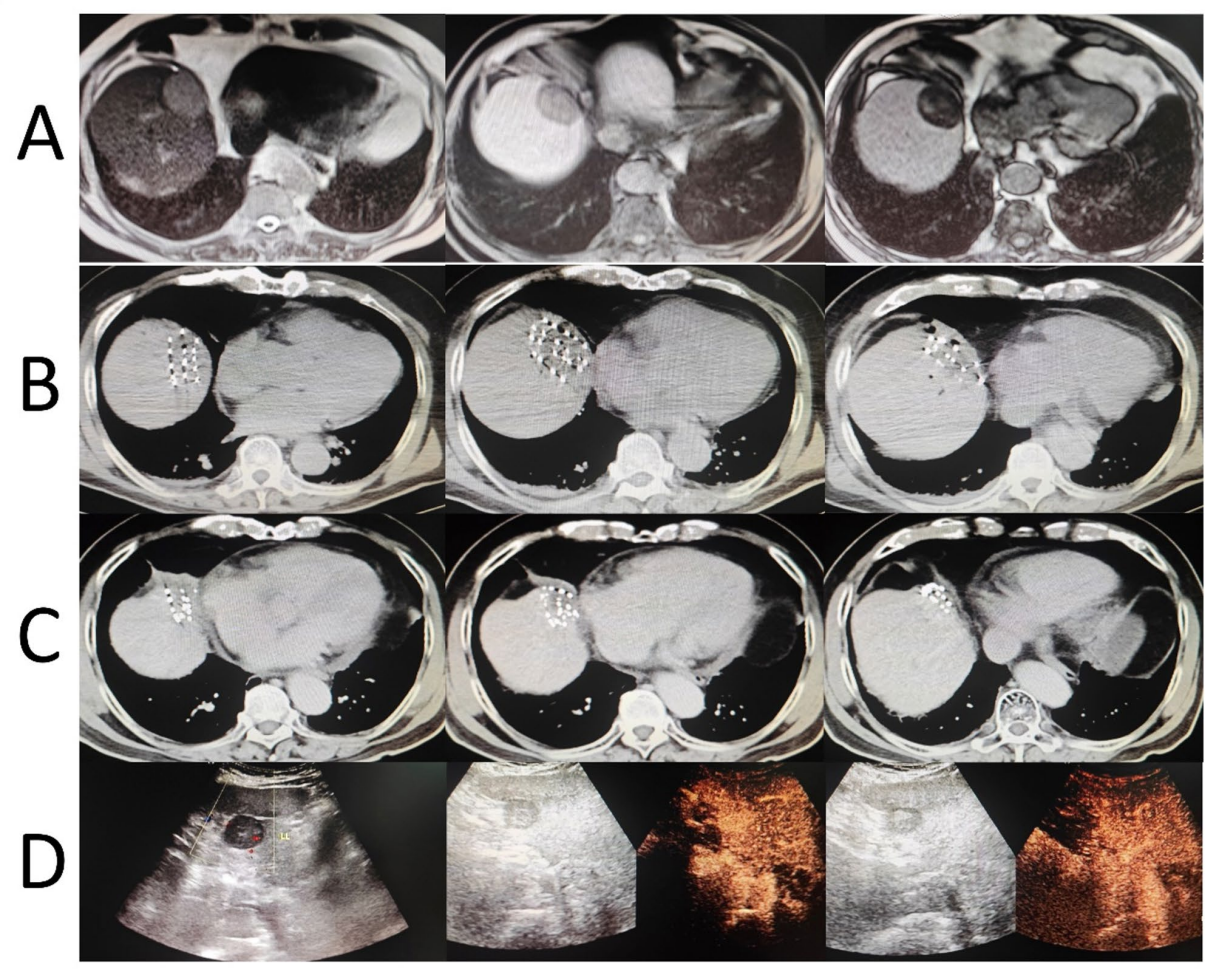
Case of a 72-year-old male patient. In January 2023, he underwent MRI (A) due to elevated alpha-fetoprotein (AFP) levels, which revealed a new hepatic lesion in segment VIII (3.6 cm) with restricted diffusion and heterogeneous enhancement. Biopsy confirmed hepatocellular carcinoma. Given the patient’s advanced age and the location of the lesion at the diaphragmatic dome, he underwent CT-guided ^125^I particle implantation for liver cancer in February 2023 (B). A follow-up CT scan six months postoperatively (C) showed significant reduction in the lesion. In May 2024, an acoustic contrast imaging of abdominal organs (D) detected a new hypoechoic lesion in the left liver (2.8 cm), suggestive of liver cancer recurrence. The patient subsequently underwent ultrasound-guided radiofrequency ablation of the hepatic tumor in May 2024. After ^125^I particle implantation, the patient achieved partial remission at three months, with a local progression-free survival (LPFS) of 15 months and an overall survival (OS) of 22 months
